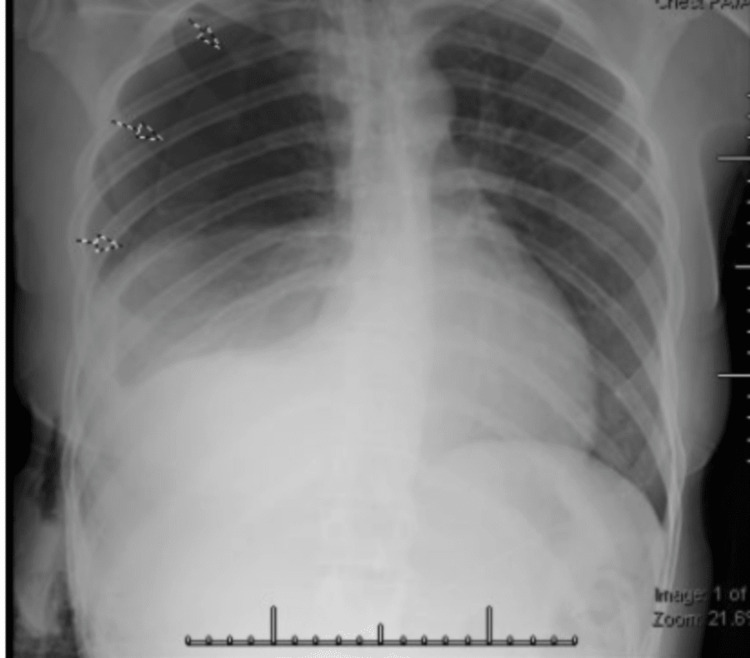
The patient's chest X-ray shows complete opacification of the right hemithorax, which is likely due to the large right pleural effusion and associated right lung atelectasis with leftward mediastinal shift.
